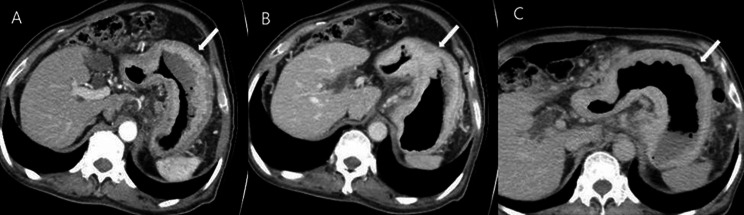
A patient with poorly cohesive type T4a stomach cancer. A and B In the arterial and portal phases, diffuse thickening of the gastric wall along the greater curvature of the gastric body is observed. This thickening exhibits a double-layered appearance, indicative of a type II positive dynamic CTTM sign, resulting from the thickened and enhanced outer low-density layer (arrow) compared to the normal gastric outer layer. C In the delayed phase, the lesion displays homogeneous transmural enhancement with perigastric fat strands, characterized as a type I positive CT transmural sign (arrow). The CTTM pattern is classified as 1-1-1
